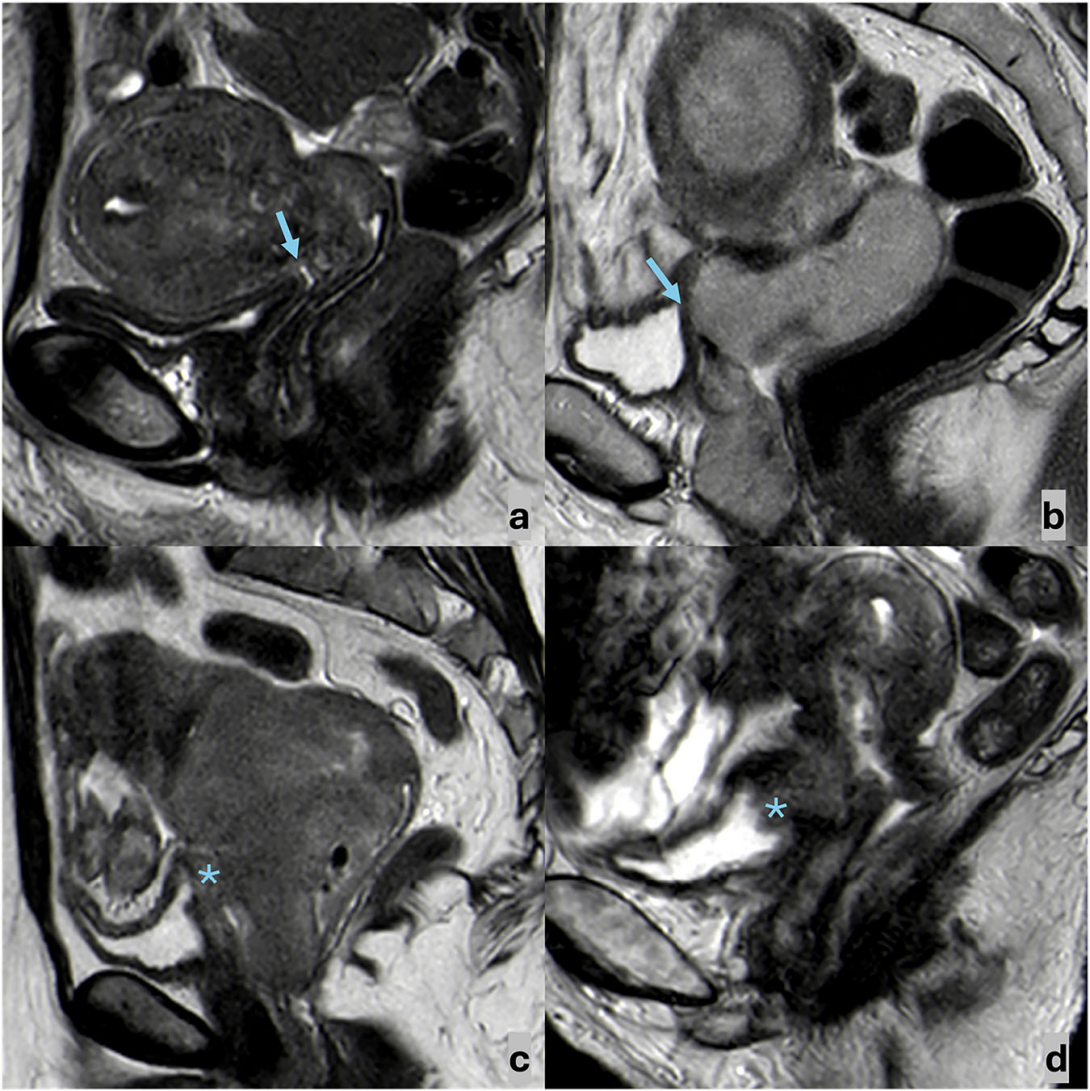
Sagittal T2-weighted FSE images showing the possible appearances of bladder wall–cervical cancer relation. a A fatty cleavage plane (arrow) may be observed between CC and the bladder, which shows a thin T2-hypointense wall. b The fatty cleavage plane between CC and the bladder is obliterated (arrow), but the bladder wall appears thin and T2-hypointense. c The fatty cleavage plane between CC and the bladder is obliterated, and the bladder wall appears thickened, with intermediate signal intensity (star). d The fatty cleavage plane between CC and the bladder is obliterated, the bladder wall appears thickened, with intermediate signal intensity, and neoplastic tissue protrudes in the bladder lumen (star)
